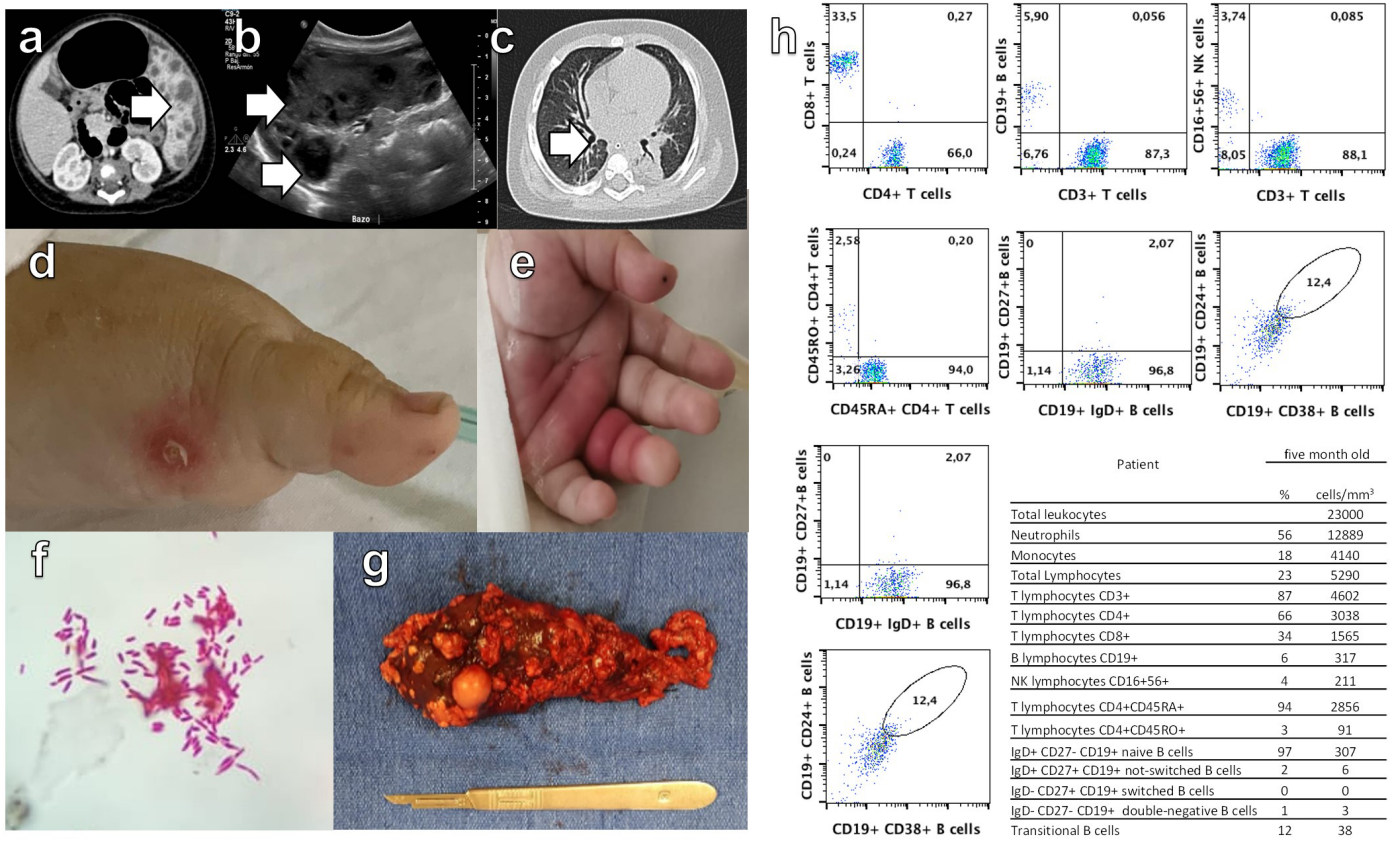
Abdominal computed tomography (CT) scan (a) and ultrasound (b), with white arrows indicating multiple abscesses. Image (c) shows a pulmonary nodule detected by CT scan. Images (d, e) display acral skin nodules. In (f), *Mycobacterium bovis* identification in blood culture is shown. Image (g) presents the macroscopic visualization of the spleen removed by surgery. Flow cytometry (h) reveals low levels of double-negative T cells, as well as decreased memory B cells without isotype switching and an absence of isotype-switched memory B cells.
